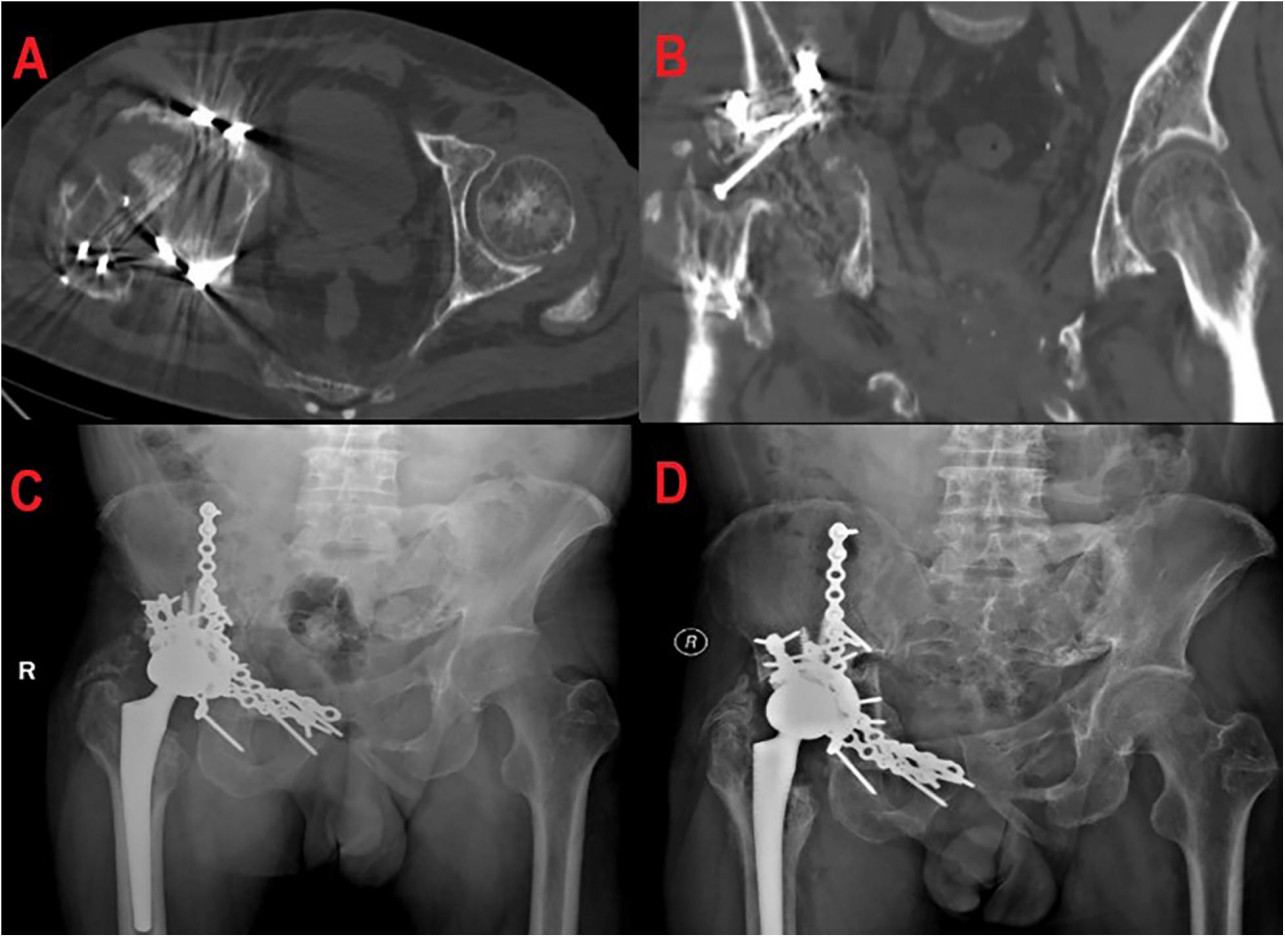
(A,B) CT showed collapse and absence of the right femoral head, suggesting osteonecrosis of the femoral head. (C) X-ray imaging after right total hip arthroplasty showed good prosthesis position. (D) The prosthesis position was still good at 30 months after operation, with no obvious loosening.
